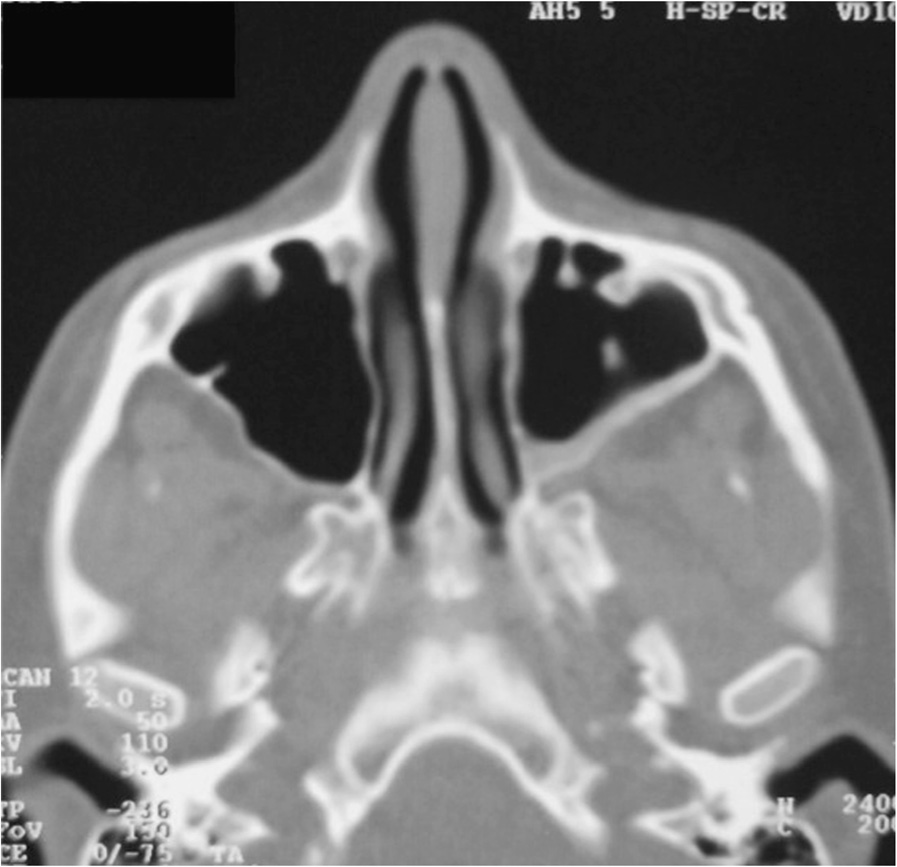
Computed tomography axial projection at 12 months.
